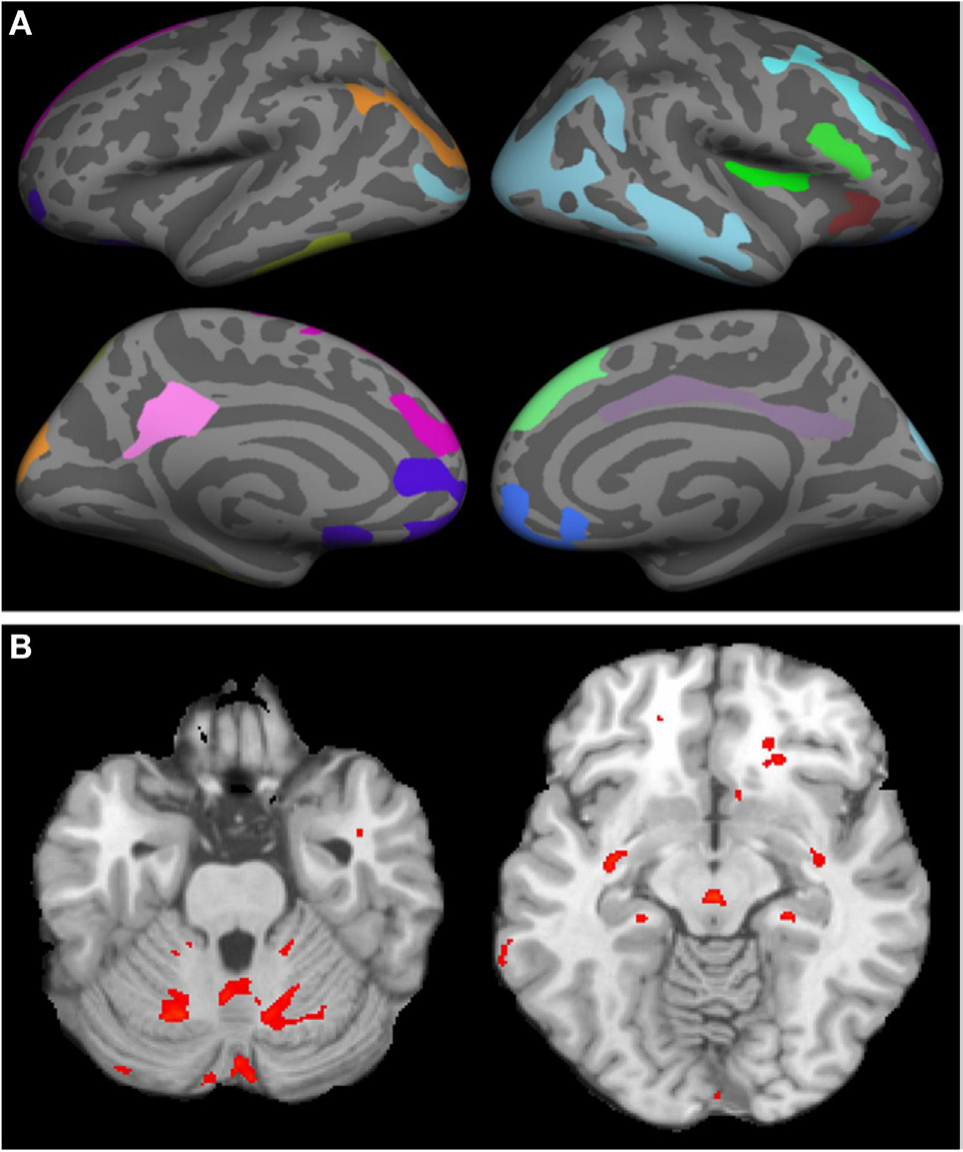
Cortical thinning and regional atrophy analyses. (A) Volumetric MRI. Left (first column) and right (second column) hemispheres showing cortical thinning in West Nile infected individuals as compared to a database of MRIs from healthy controls with lateral (top row) and medial (bottom row) views. Colored regions indicate areas of thinning. In the left hemisphere, parts of the posterior cingulate cortex (pink), parts of the superior frontal cortex and medial-orbito frontal region (magenta), anterior cingulate cortex and inferior frontal cortex (violet blue), parts of the cuneus (orange) and para hippocampal region (pale green) were identified. In the right hemisphere, parts of the middle and inferior temporal cortex, and supramarginal region (light blue), inferior frontal region and insular cortex (bright green and red), parts of the superior frontal cortex (light green, blue, and violet), cingulate cortex (light purple), and inferior frontal region (blue) were identified. (B) Tensor-based morphometry (TBM) analysis of regional atrophy. Regions with regional atrophy as detected by TBM are identified in red.
